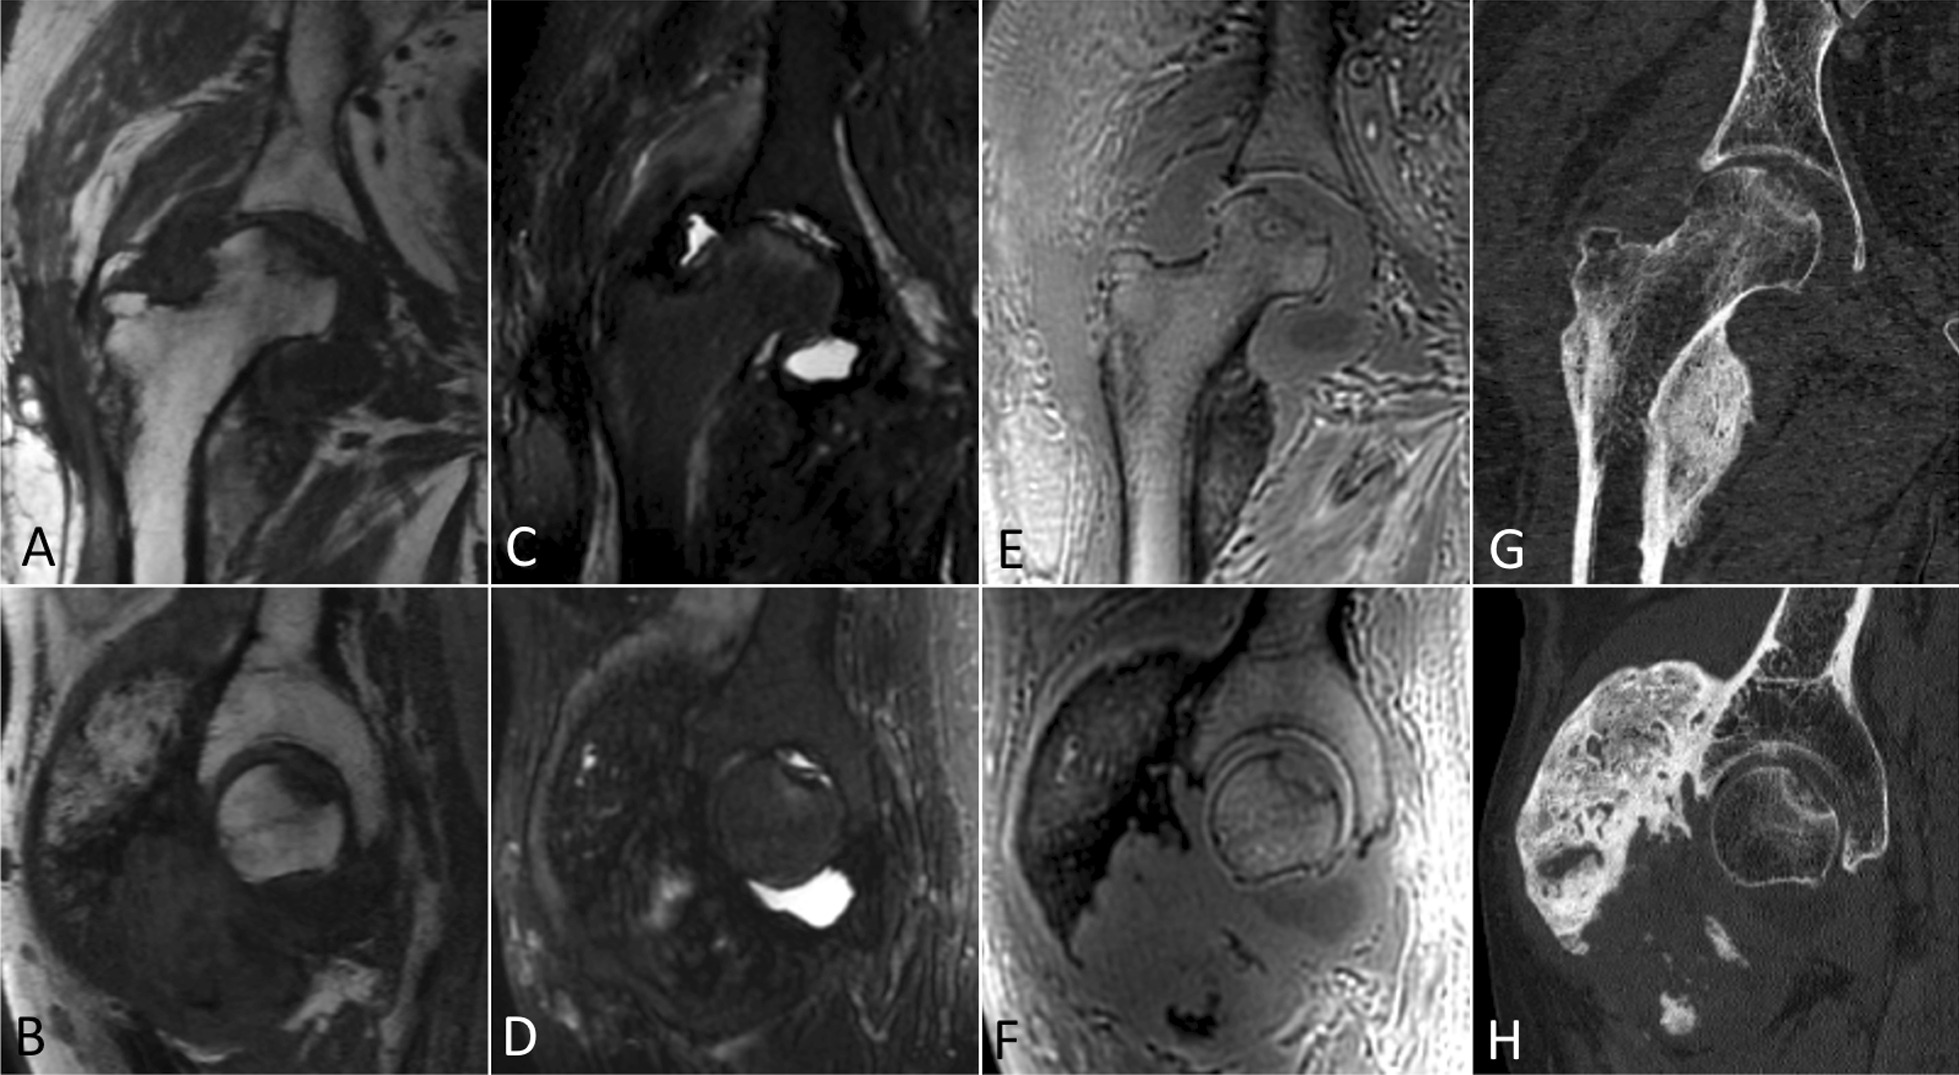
Osteonecrosis of the femoral head in a patient with an anterior NHO of the right hip. Coronal and sagittal images in T1-weighted (A, B), STIR (C, D), and ZTE (E, F) sequences and in CT (G, H)
